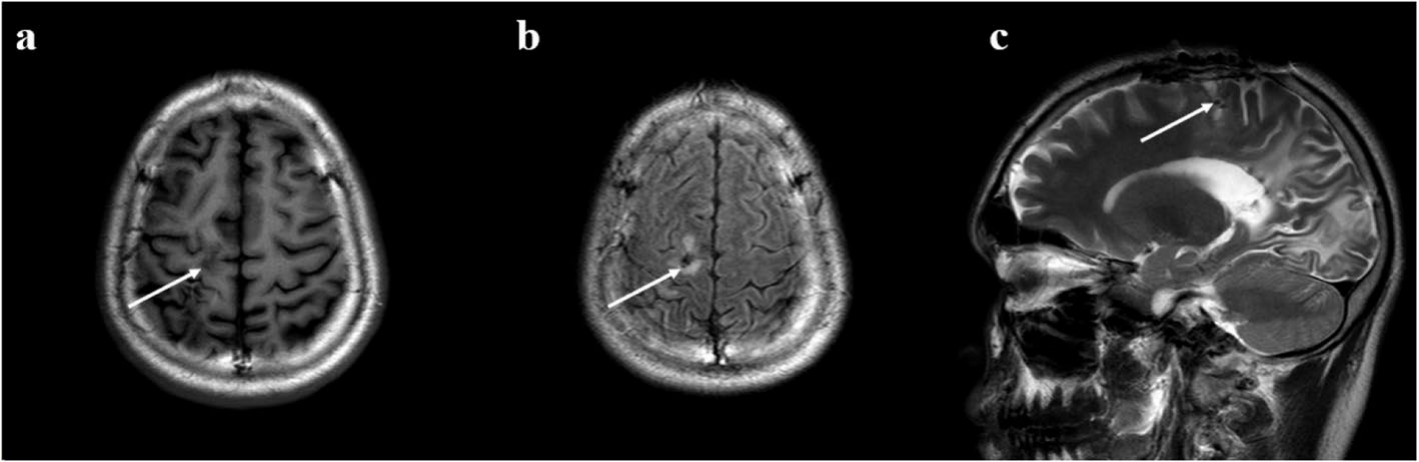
Postoperative follow-up brain MRI scans
